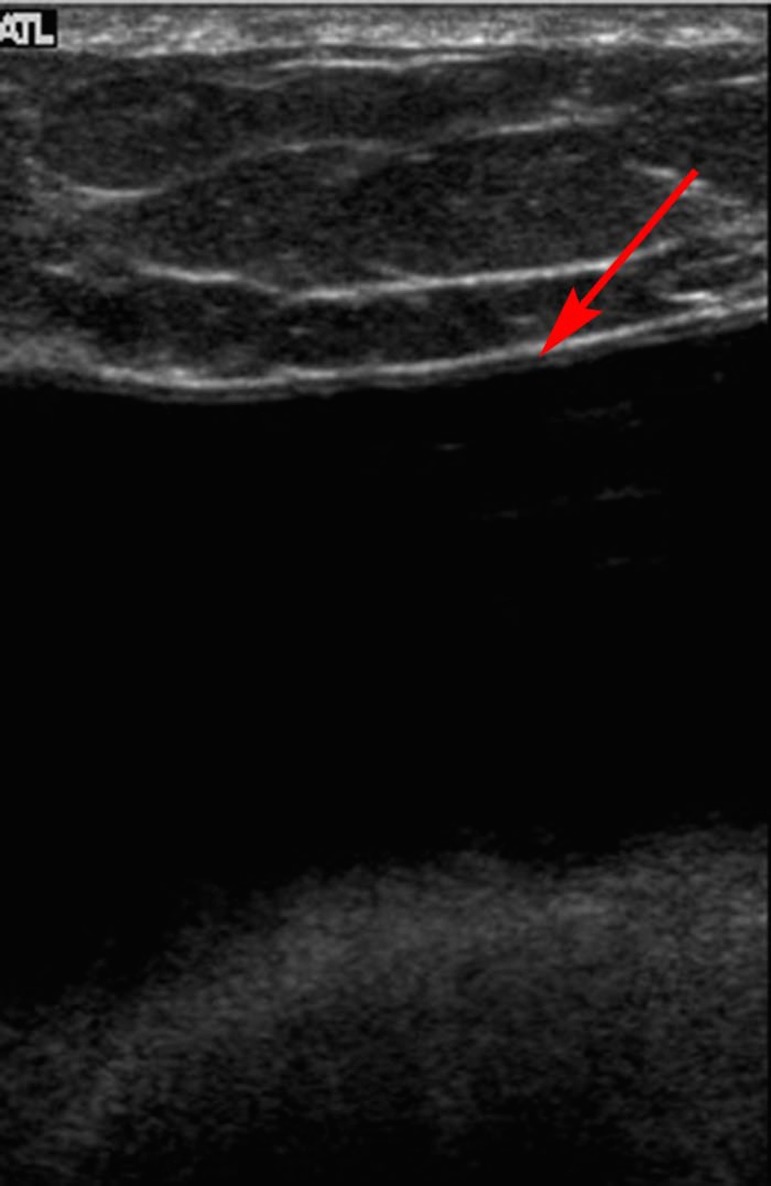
Gray scale ultrasound image of the right breast A simple fluid collection (red arrow) is seen superficial to the underlying implant.
